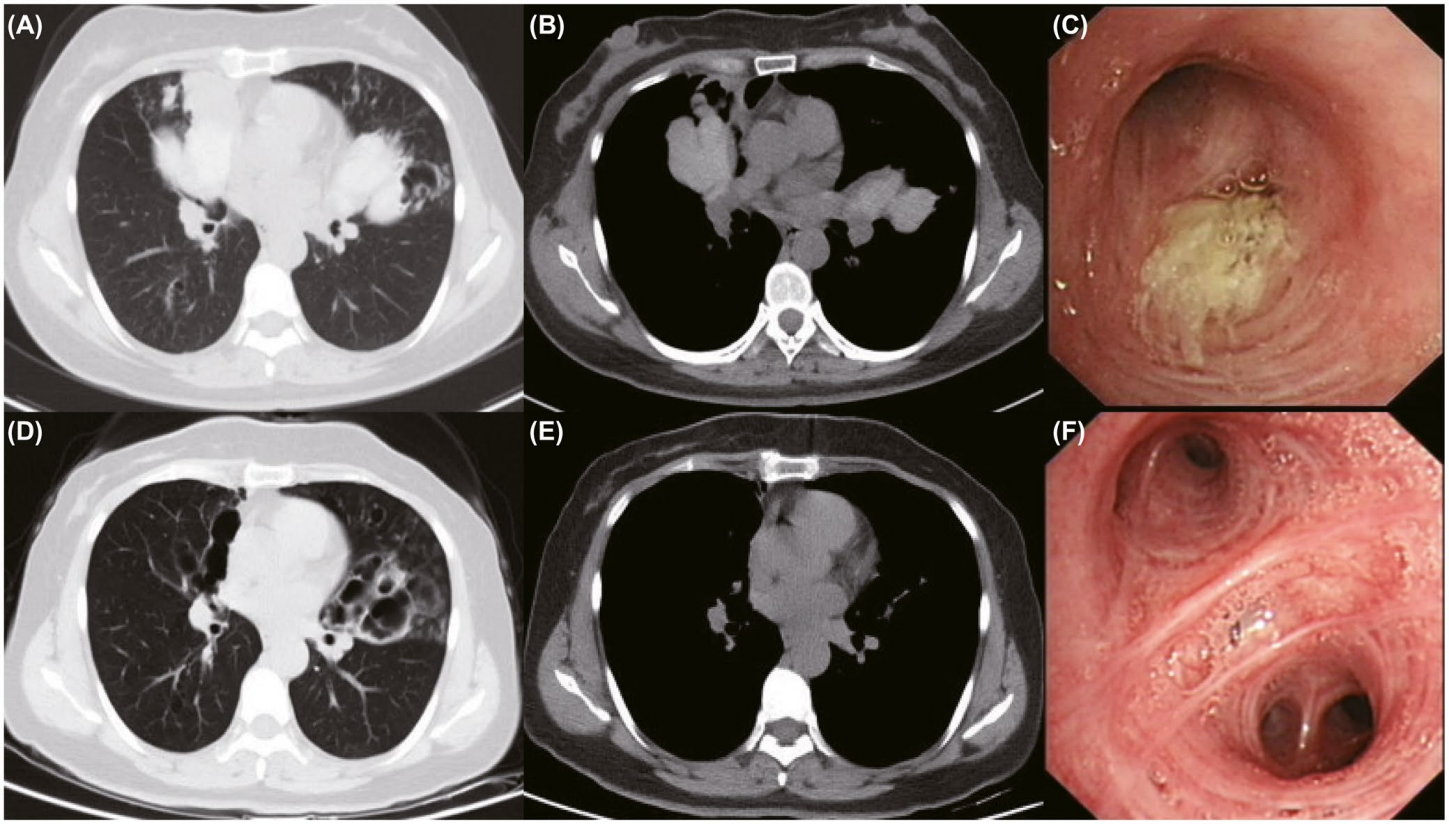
CT scans and bronchoscopy images of HAM before and after the comprehensive treatment. (A,B,C): before the treatment. (D,E,F): after the treatment
